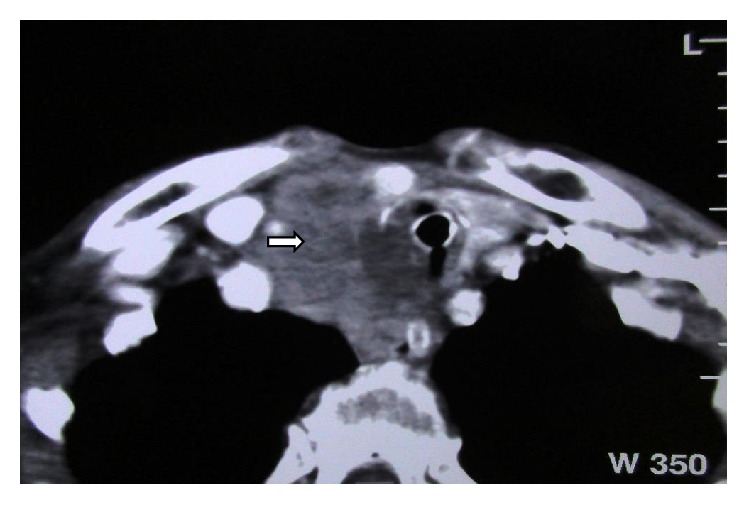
Computed tomography showing the cervical CASTLE tumour surrounding the trachea anterolaterally and invading the thyroid gland.
